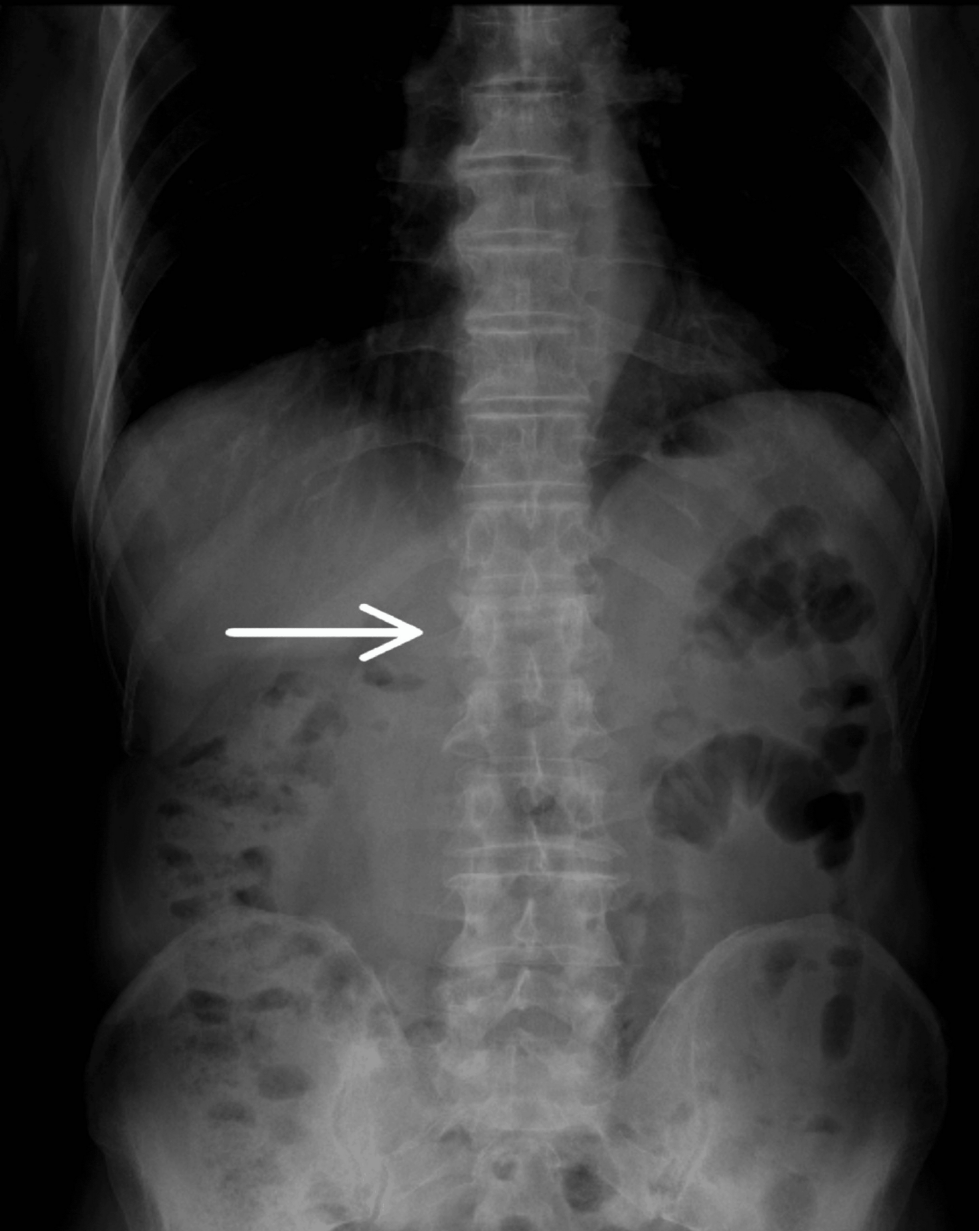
Anteroposterior X-ray view showing L1 vertebral fracture.
